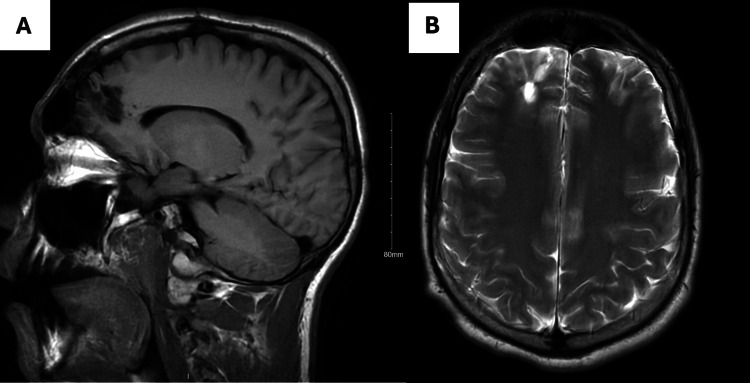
Postoperative MRI of the brain at six-month follow-up The sagittal view (A) and axial view (B) of the T2-weighted magnetic resonance imaging with contrast six months postoperatively, revealing postsurgical changes at the site of the right frontal lobe craniotomy with encephalomalacia.
